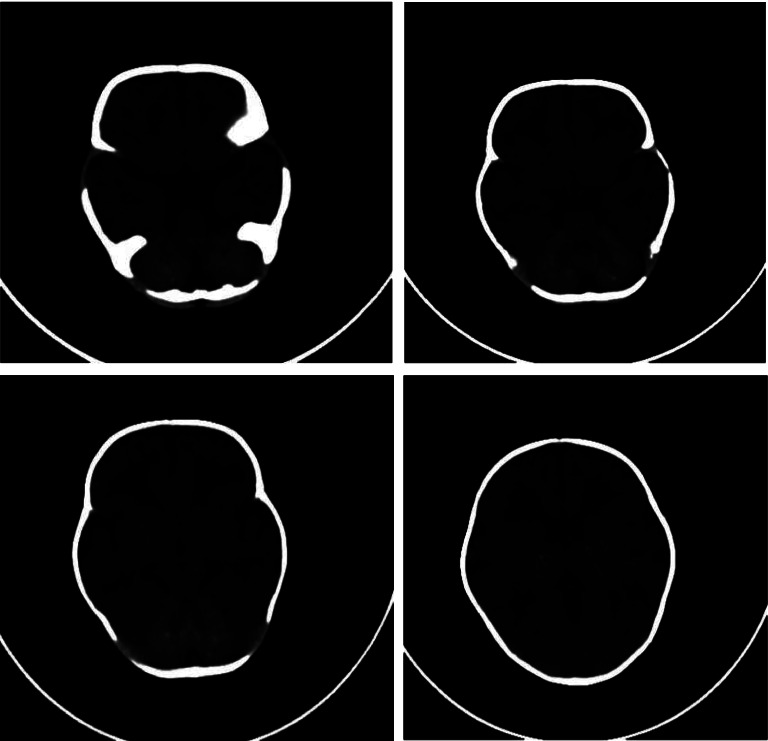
Brain CT scan: diffuse calcifications involving both lobes as well as basal ganglia and extensive bilateral periventricular hypodensities.
